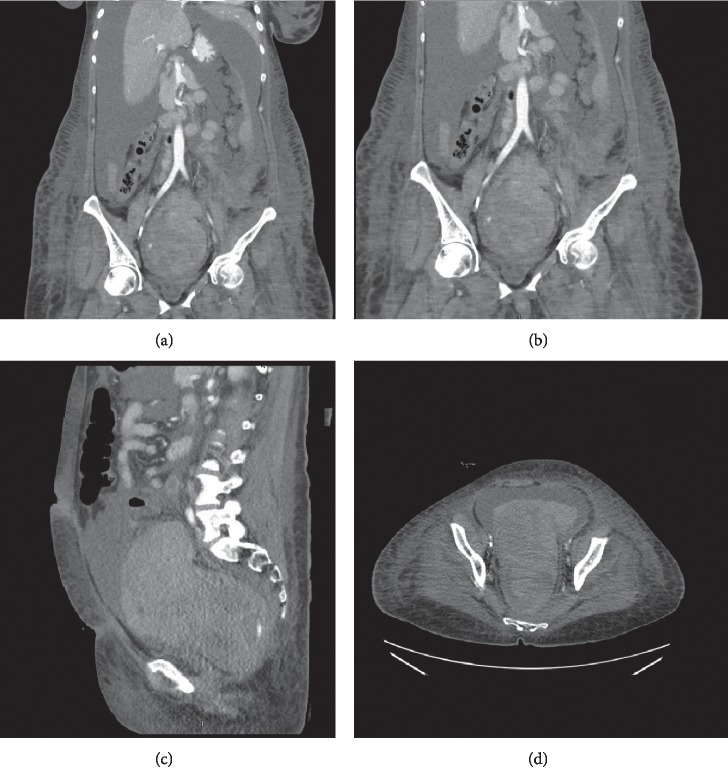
a, b) Coronal views of CT abdomen and pelvis with contrast show a heterogeneous, mass-like appearance of an organized rectal hematoma. (c) Sagittal view shows prominent rectal dilatation with high intensity signal showing probable active contrast extravasation. (d) Axial view of the hematoma.
